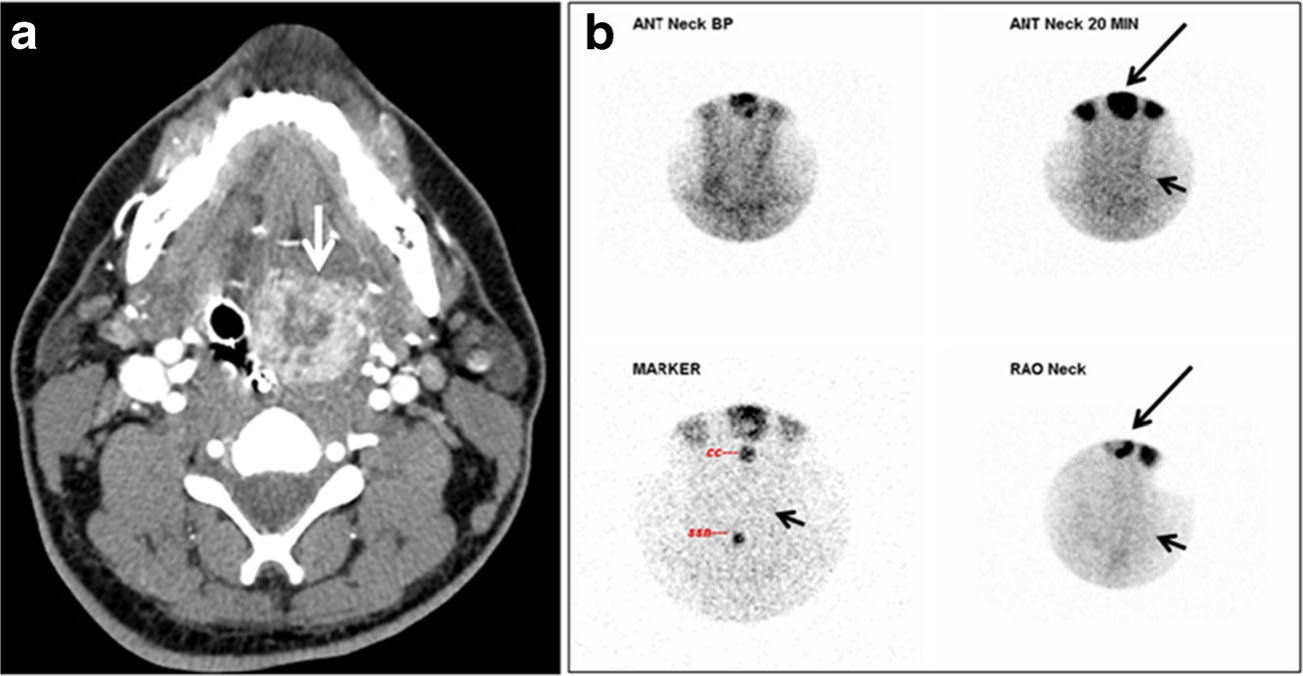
Lingular thyroid in a 33-year-old male who presented with oropharyngeal bleeding. a Axial enhanced neck CT scan at the level of mandible demonstrates a 3 × 3 × 3.4 cm round, partly well-delineated, heterogeneously enhancing lesion (*white arrow*). It is predominantly on the left side of the oropharynx and to some extent at the mid part of the base of the tongue. Thyroid gland was normal (not shown). b Image of the anterior face and neck taken 20 minutes after Tc99m-Pertechnetate injection shows absent thyroid radiotracer uptake in normal thyroid anatomical location (*black short arrows*). There is an area of increased uptake (*long black arrows*) corresponding to posterior tongue mass identified on CT scan
